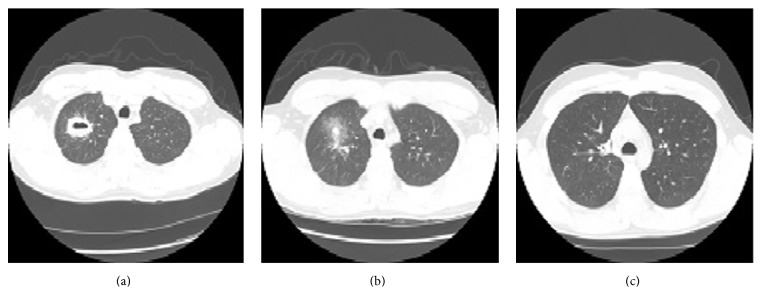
(a) Chest axial view of CT performed on the day of admission, showing a thick-wall cavitary lesion (6.0 × 1.6 × 3.5 cm) in the right upper lobe of the lung. (b) Chest axial view of CT performed at 2-month follow-up, showing foci with ground glass opacity in the anterior right upper lobe of the lung surrounding a 9.9 mm nodular density. (c) Chest axial view of a CT performed at 3-month follow-up, showing complete resolution of the right upper lobe cavitary lesion.
